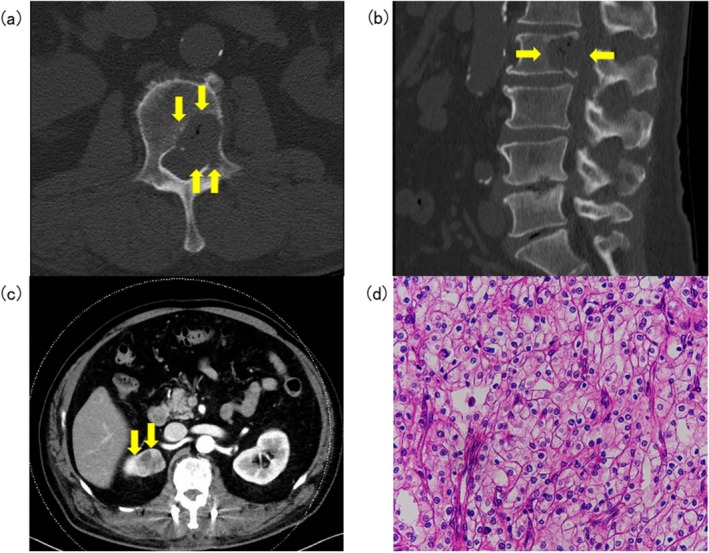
Initial diagnostic imaging of the patient. CT revealed bone mass in the L2 vertebra. (a) Coronal plane. (b) Sagittal plane. (c) Contrast‐enhanced CT revealed a mass in the upper pole of the right kidney. (d) Pathological examination of the resected tumor revealed clear cell renal cell carcinoma.
